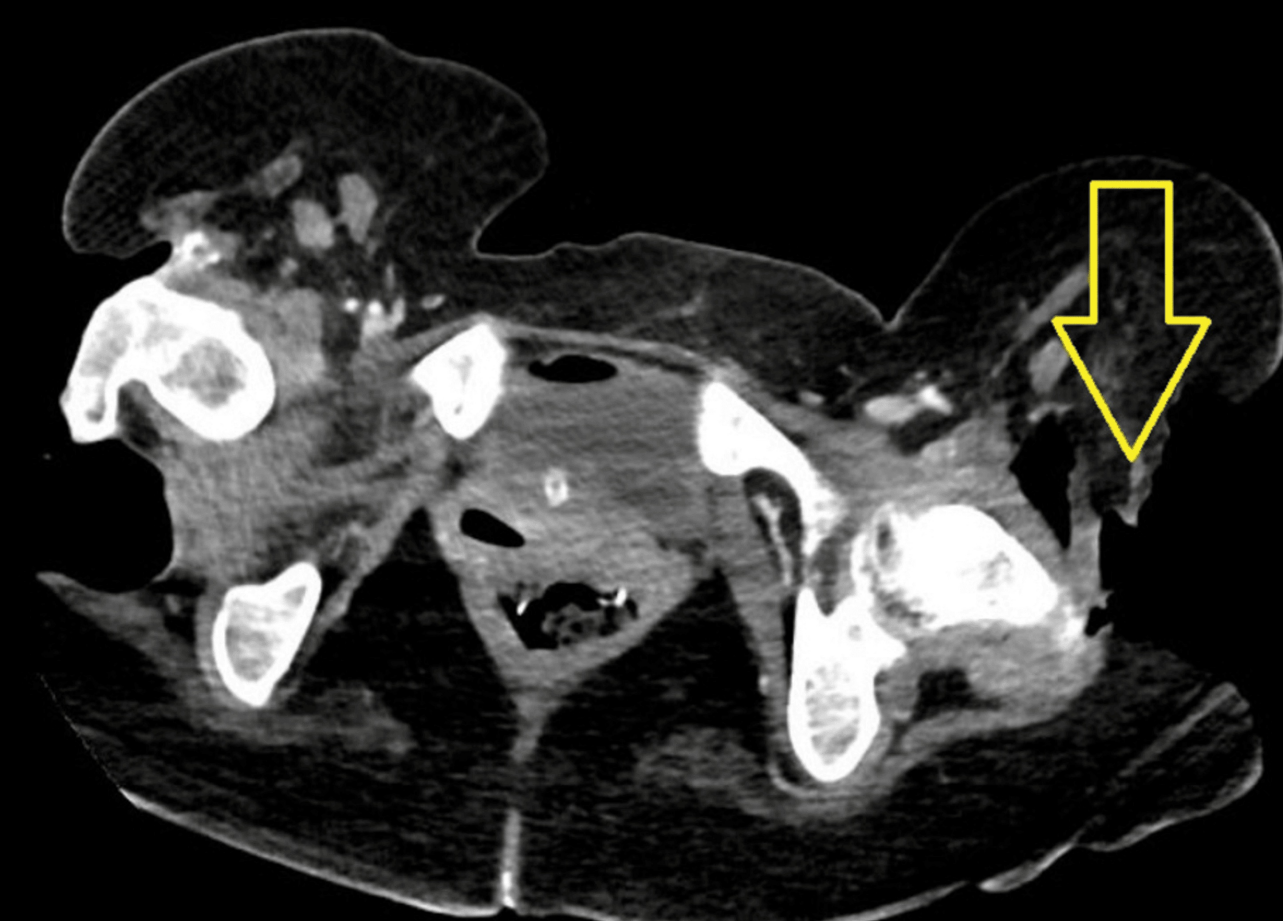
CT bilateral lower extremities with IV contrast revealing a large wound of the left thigh, subcutaneous emphysema tracking inferiorly along the gluteus musculature and quadriceps musculature (yellow arrow). There is exposed bone along the greater trochanter, with thinning and heterogeneity of the cortex of the exposed bone. Minimal surrounding stranding of the soft tissues.
